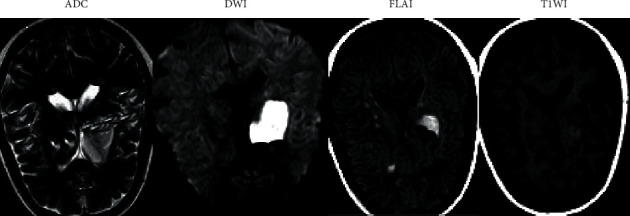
MRI images of early acute ischemic stroke.
